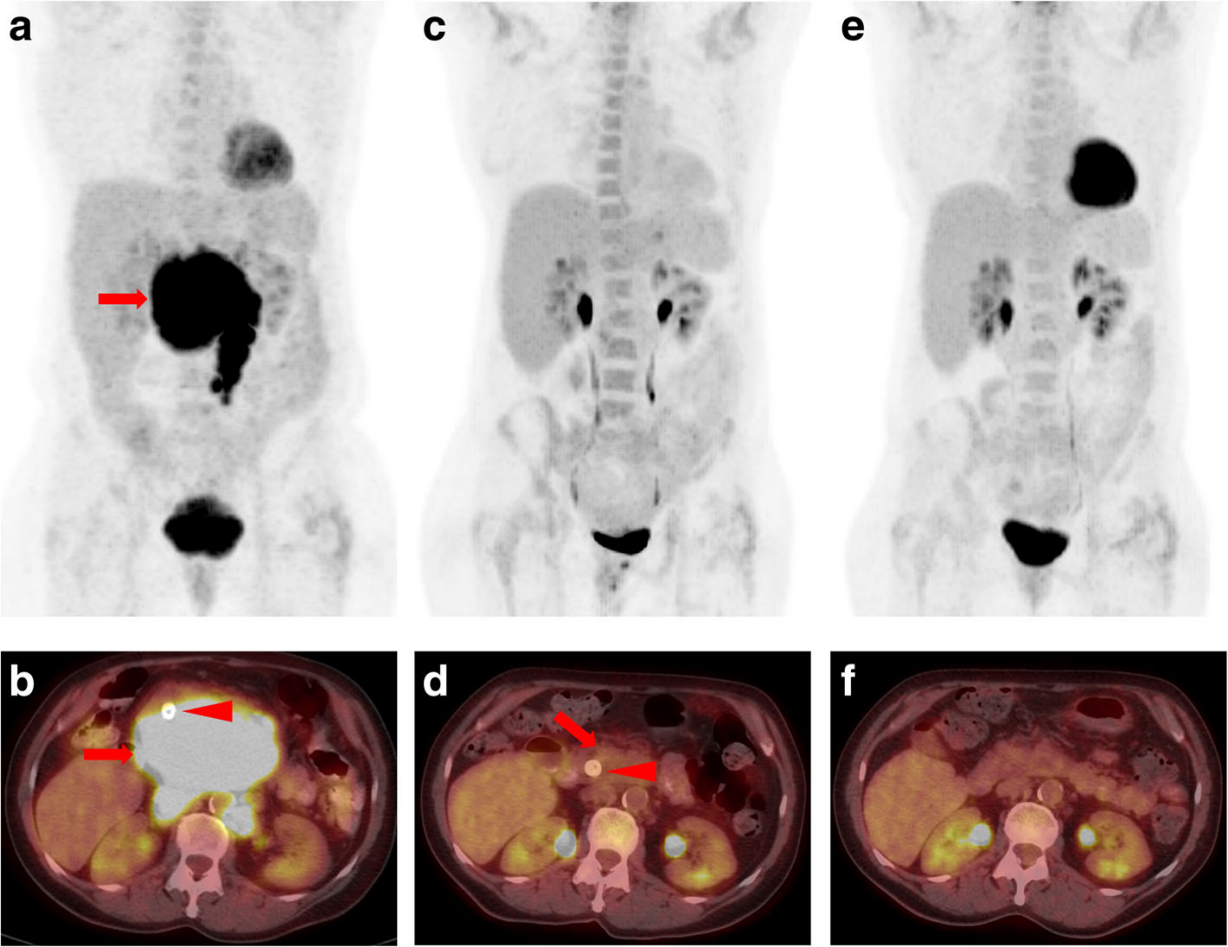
a Baseline whole-body MIP image demonstrating intense FDG uptake in a large retroperitoneal mass (red arrow) compatible with patient’s follicular lymphoma. b Representative axial PET/CT image from the same time point as in (a) showing the large, FDG-avid mass (red arrow). Note the common bile duct stent (red arrowhead) that is markedly anteriorly displaced by the lymphomatous mass and explains the patient’s presentation with obstructive jaundice. c Whole-body MIP image following three cycles of R-CHOP shows no residual metabolically active lymphoma. d Representative axial PET/CT image from the same time point as in (c) is notable for the presence of minimal residual abnormal soft tissue in the retroperitoneum (red arrow, Lugano 2), with uptake equal to blood pool, compatible with a complete metabolic response. The common bile duct stent is in near-orthotopic location now that the retroperitoneal mass has dramatically reduced in size (red arrowhead). e Whole-body MIP image at the end of therapy, again demonstrating no metabolically active tumor. f Representative axial PET/CT image from the same time point as in (e) again depicts the complete metabolic response (Lugano 1) and also the removal of the common bile duct stent
